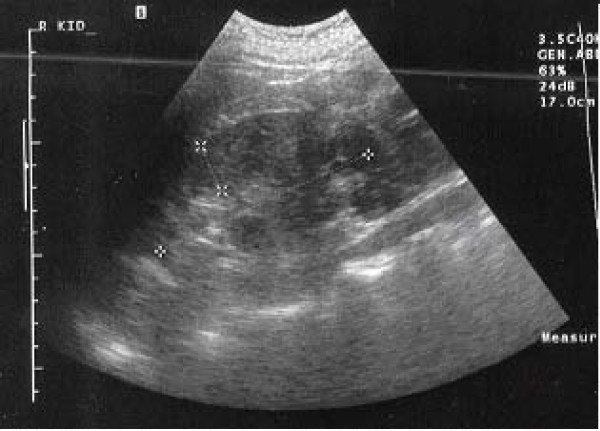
Cystic nephroma: Renal ultrasound image.
